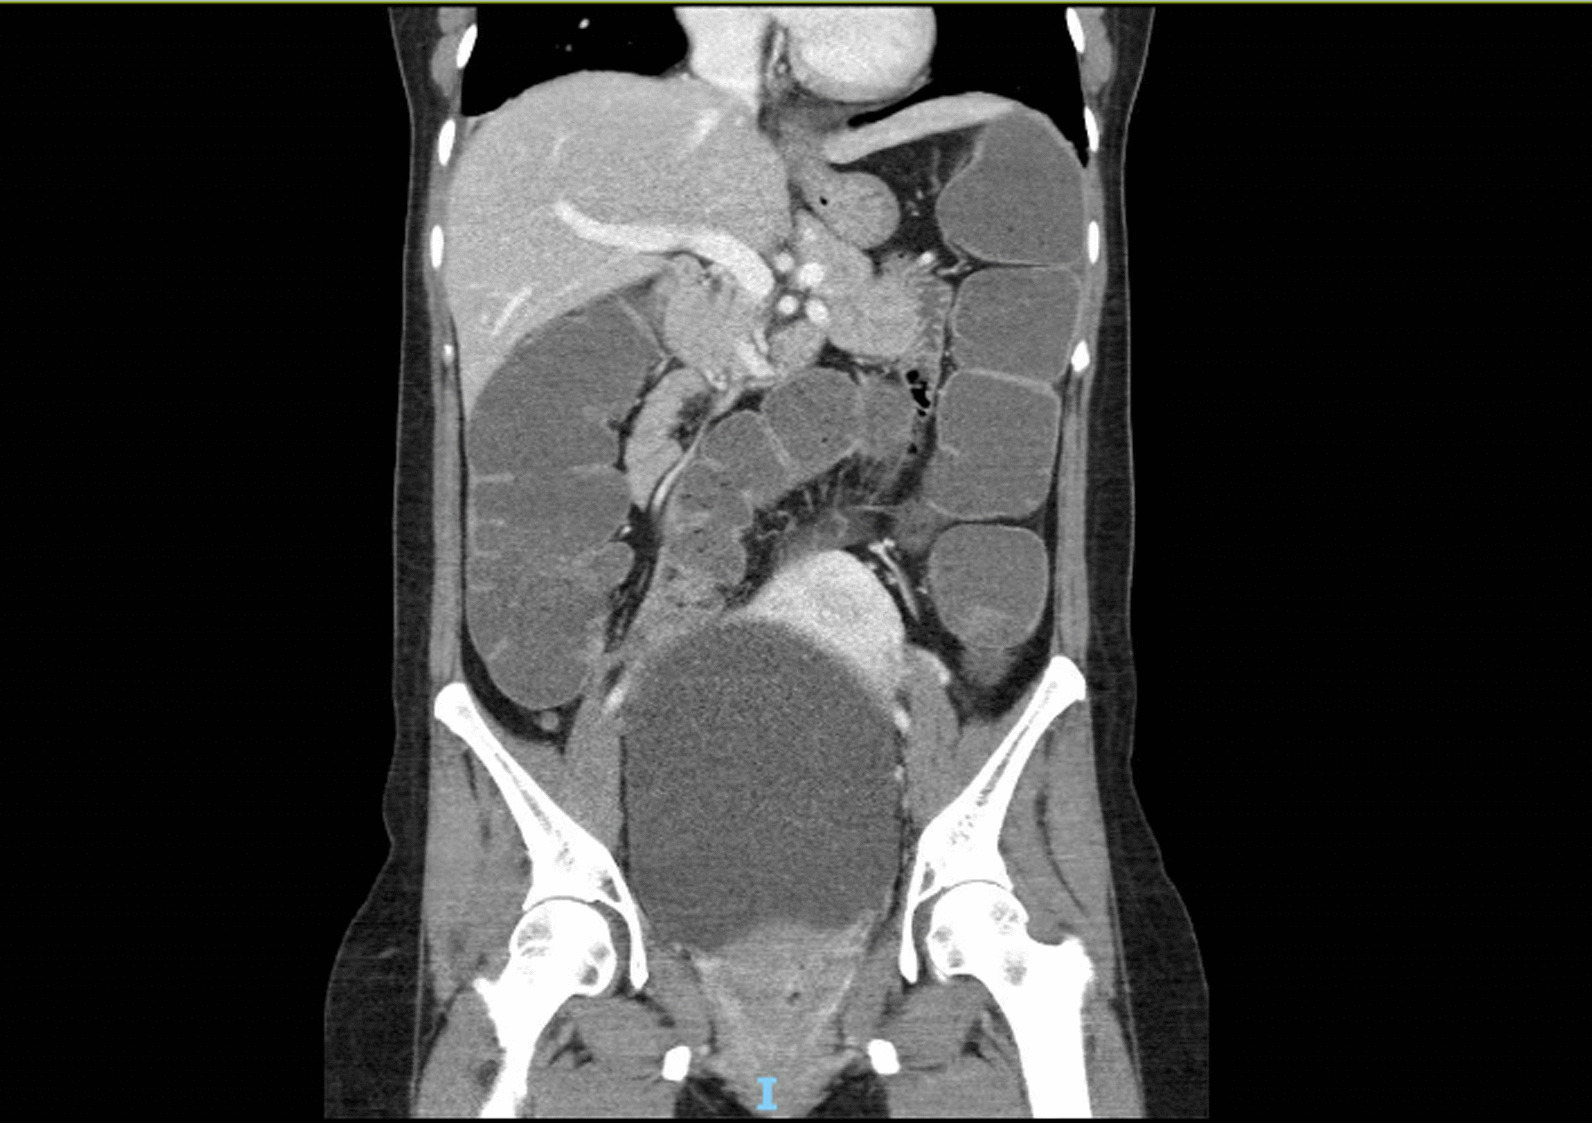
Patient’s abdomen/pelvis Computed Tomography (CT) with contrast showing dilated large bowel with transition point within the pelvis in the distal sigmoid colon, likely secondary to extrinsic compression from the distended bladder resulting in mechanical large bowel obstruction (coronal view)
